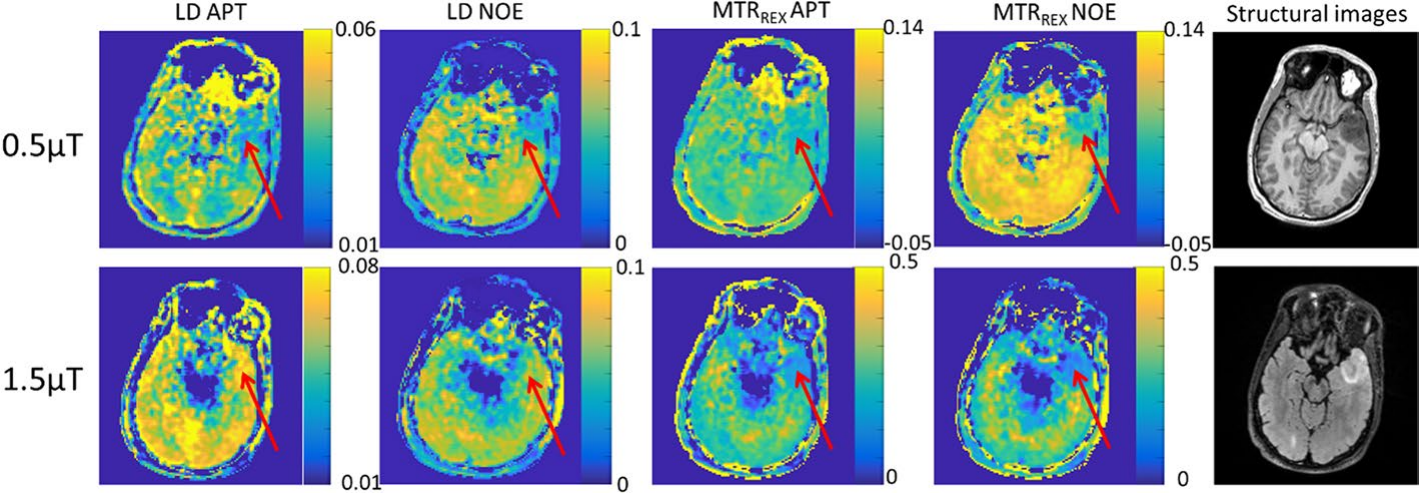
Example brain slice of patient 3 (first row: LD APT, LD NOE, MTR_REX_ APT, MTR_REX_ NOE, under 0.5 µT and T_1_ pre-contrast image; second row: LD APT, LD NOE, MTR_REX_ APT, MTR_REX_ NOE under 1.5 µT and FLAIR image
